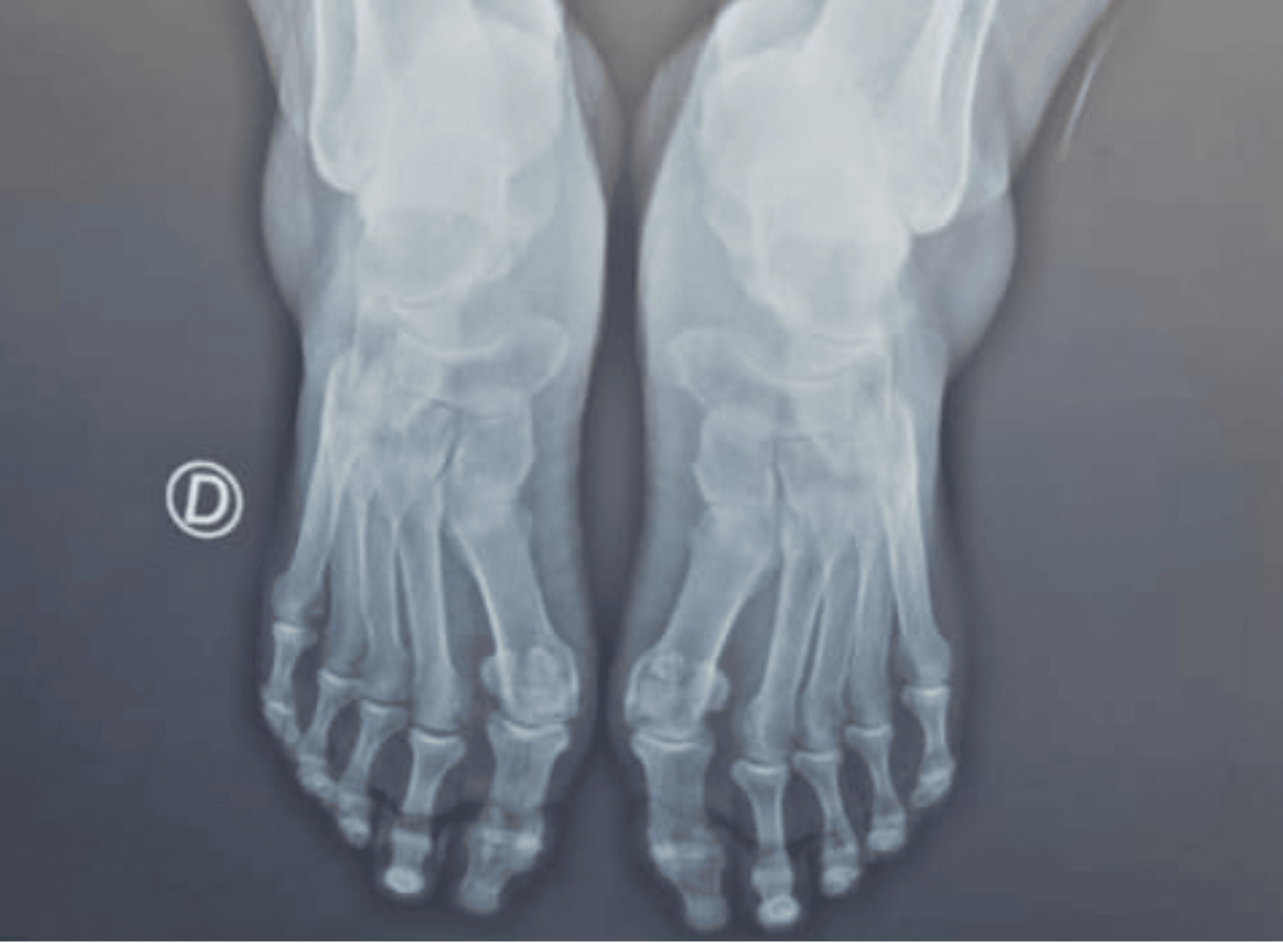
Weight-bearing dorsoplantar X-ray of the forefoot D: right foot
